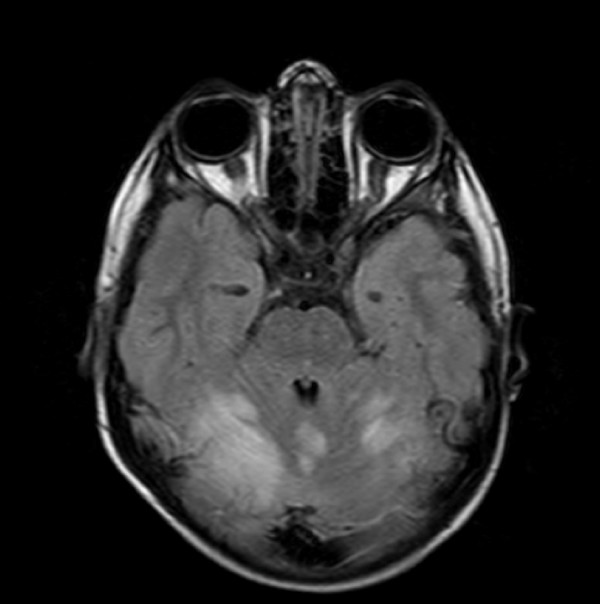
MRI-flair axial study of the brain (patient #1) showing cortical and subcortical spots with a mild mass effect, with major spreading in the cerebellum (right hemisphere > left hemisphere).
